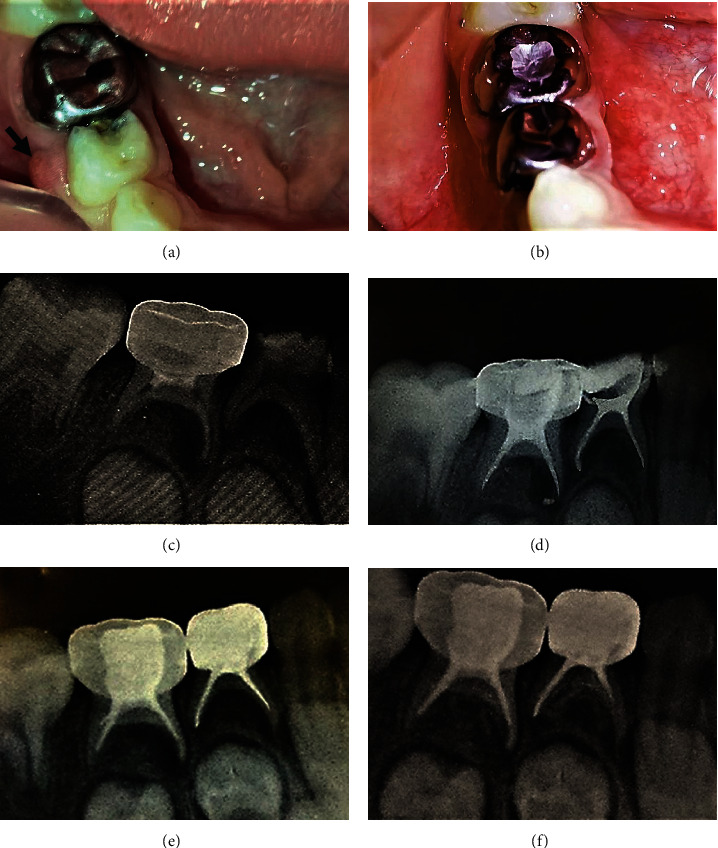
Molars (84 (an infected tooth with discharge fistula)—85 (previous failed pulpotomies tooth)) of a 6.5-year-old girl were treated with Ginge-Cal obturation material. (a) Pretreatment photo the black arrow indicates to fistula. (b) After 12 months of obturation photo (c) pretreatment X-ray, (d) postobturation X-ray, (e) after 6 months of obturation X-ray, and (f) after 12 months of obturation X-ray.
